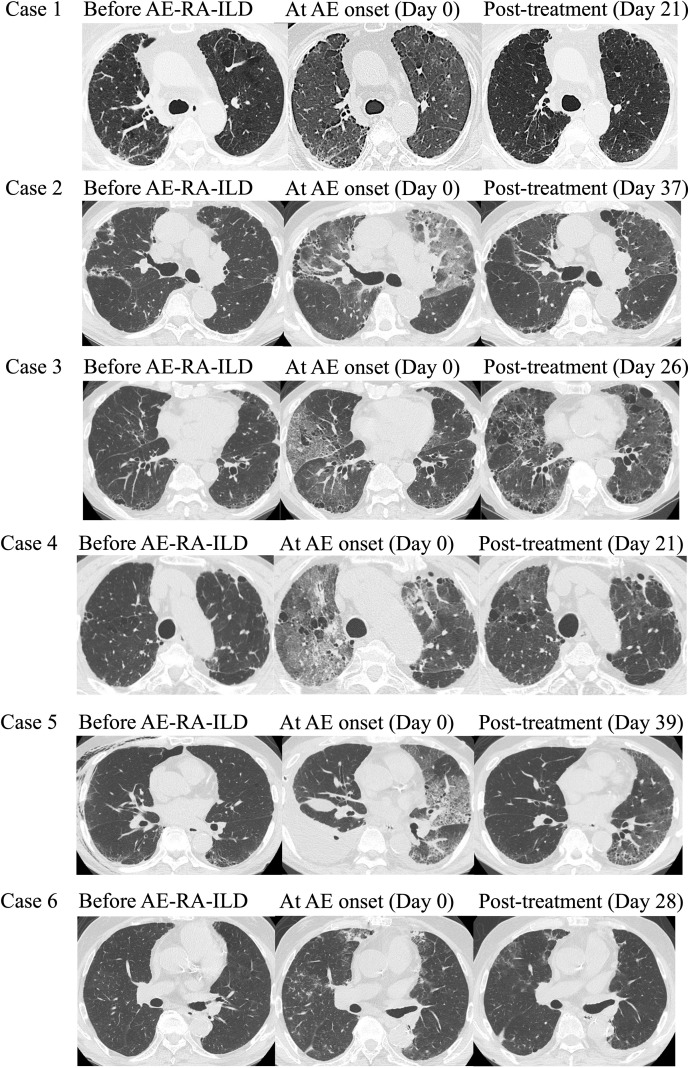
High-resolution computed tomography (HRCT) findings before rheumatoid arthritis–associated interstitial lung disease (AE-RA-ILD) onset, at exacerbation onset, and post-treatment.
